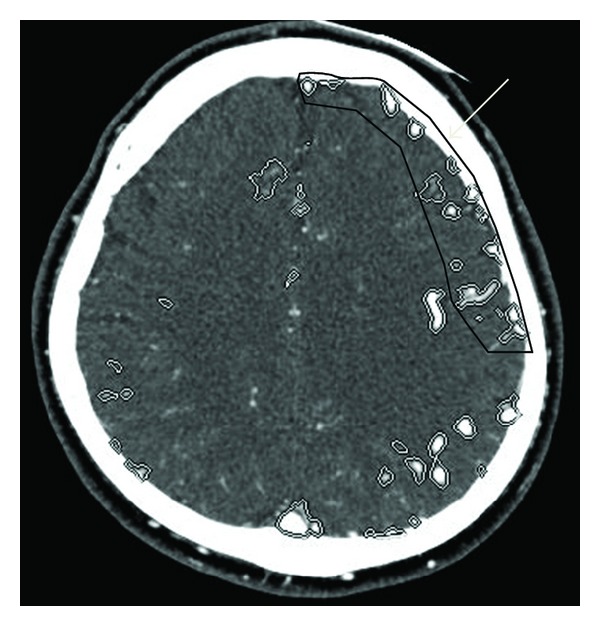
In a former axial image of 3D-CT angiography arterial phase obtained after admission, abnormal vessels (circle markings) appeared in the left cerebral hemisphere including the DLPFC (arrow).
